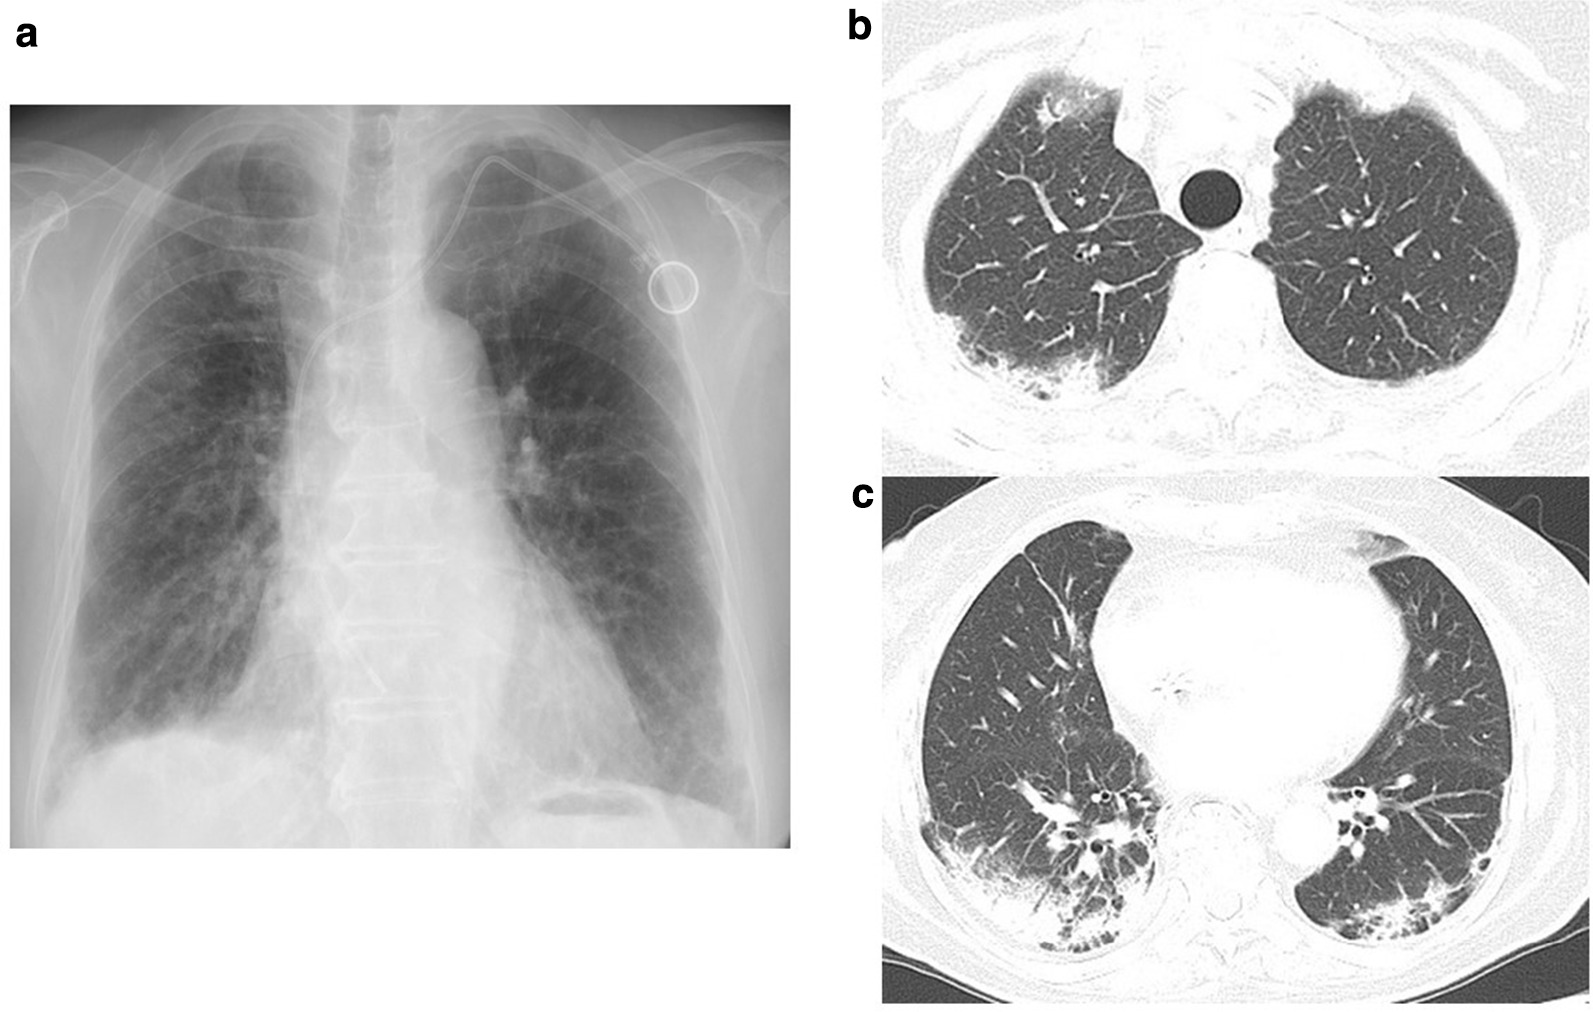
Chest x-ray and thoracic computed tomography (CT) on admission. a Chest x-ray shows bilateral, multiple-nonsegmental consolidation. b, c Thoracic CT performed at the same time shows areas of consolidation, predominantly in the subpleural areas.
